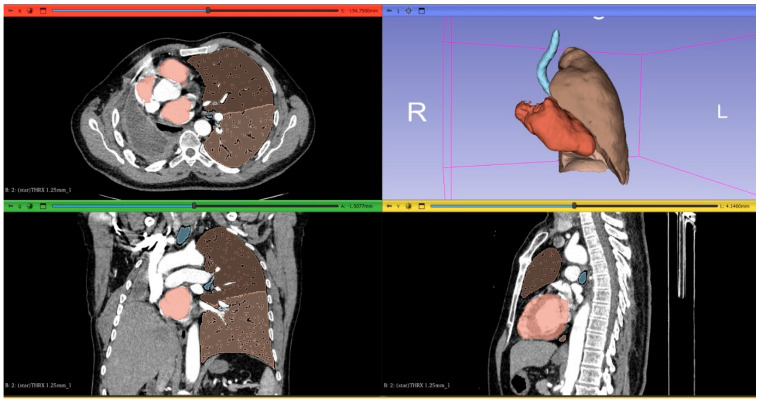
Measurement and visualization of the volume of the residual lung by 3D−CT volume measurement method with the control thorax CT of a patient who underwent pneumonectomy 1 year later.
